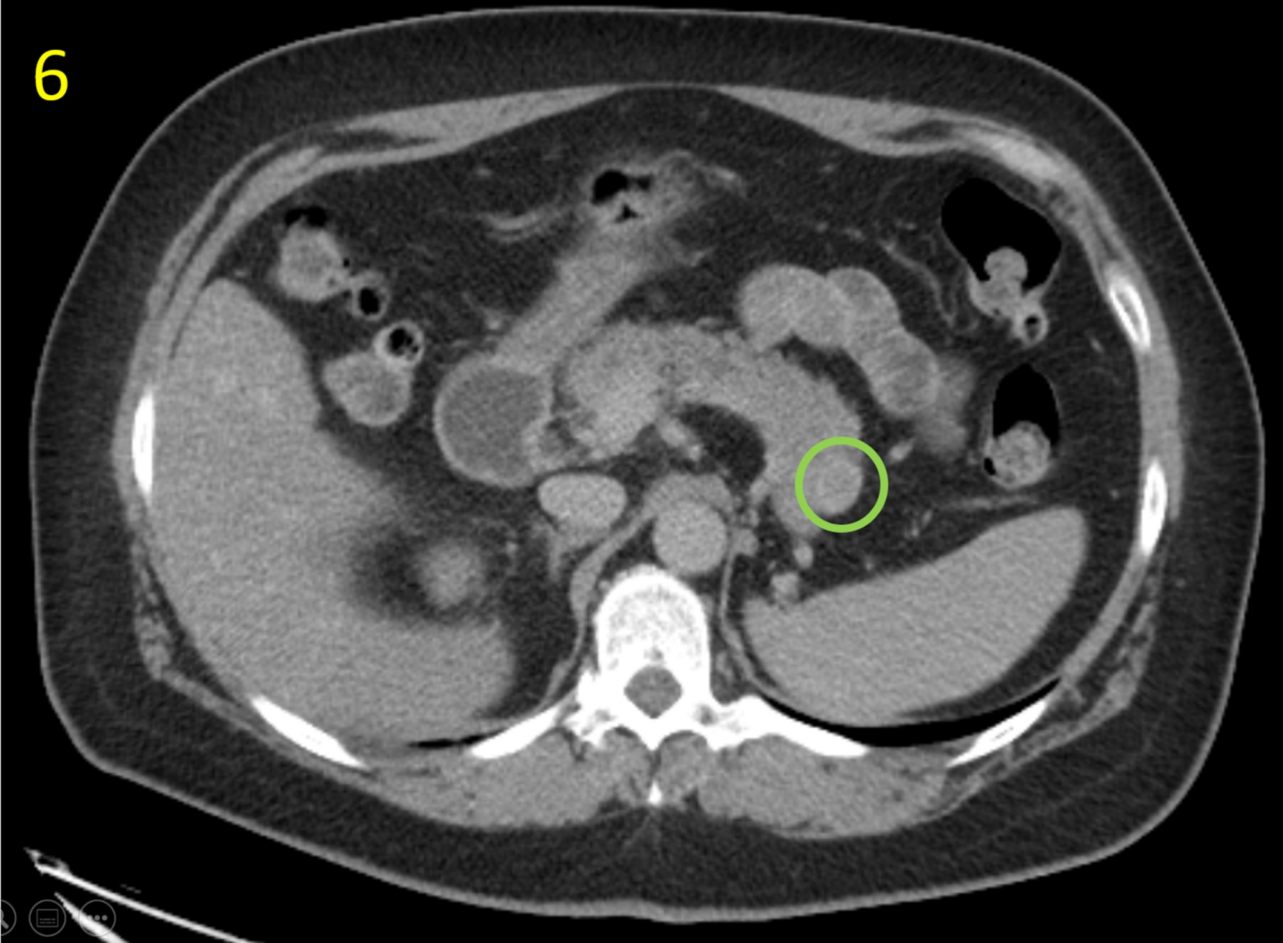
Non-Contrast Abdominal CT of Pancreas This abdominal CT demonstrates a mass suspicious for metastasis in the tail of the pancreas (green circle). The study was limited due to lack of intravenous contrast.
